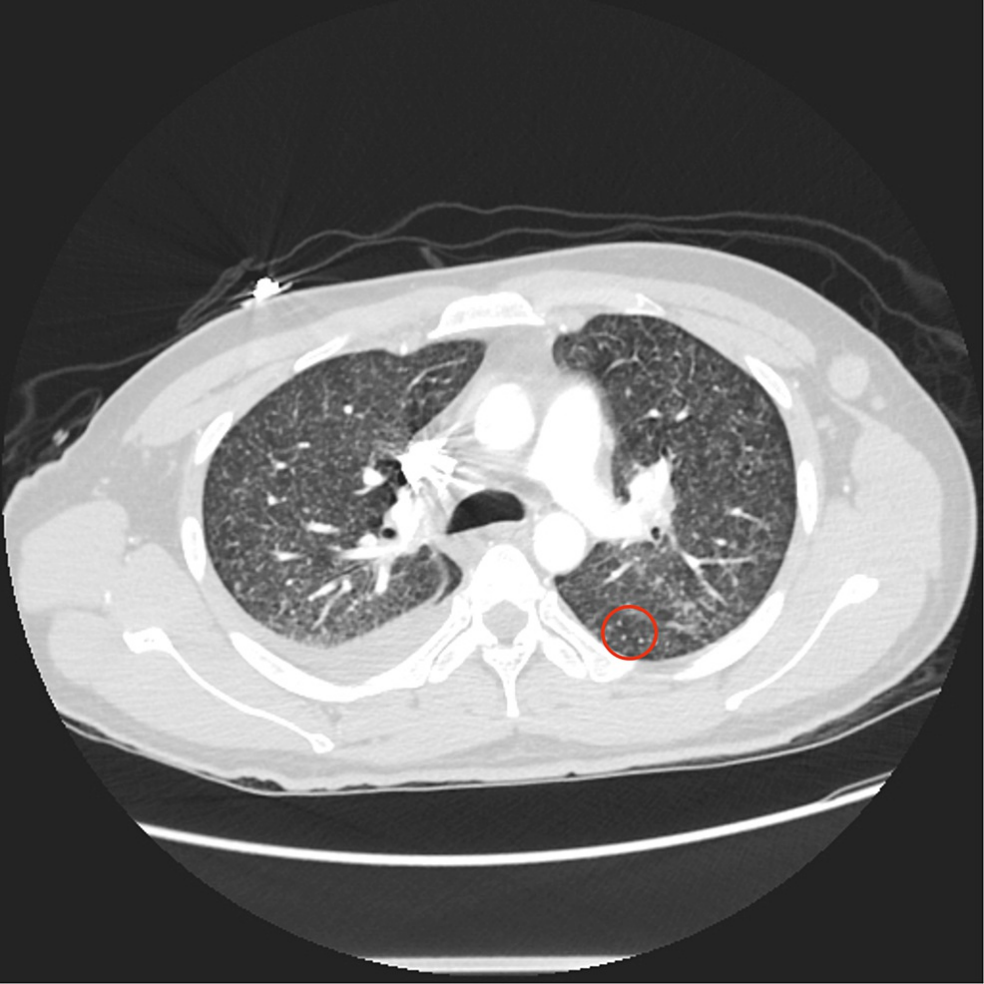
Chest CT with angiography revealing bilateral pulmonary miliary nodules (red circle)
